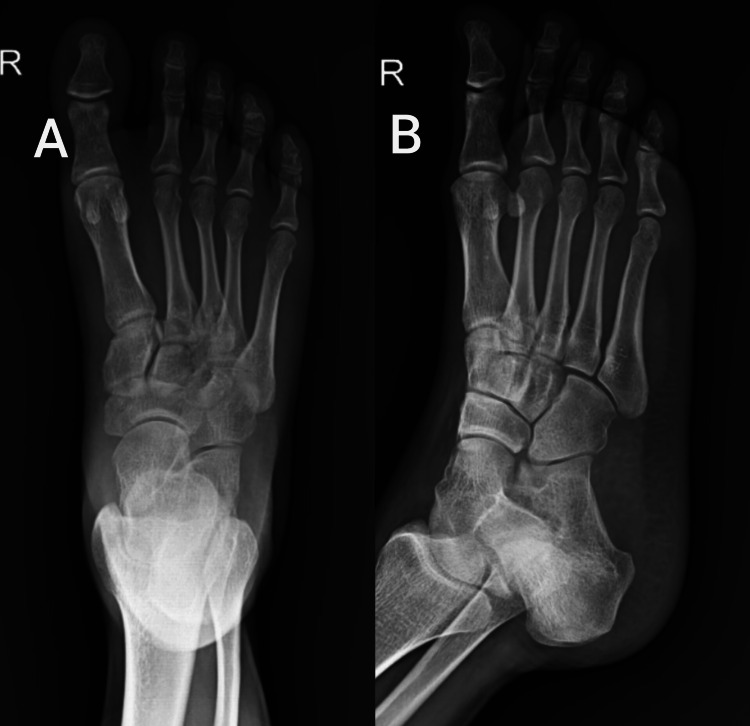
X-ray of the right ankle joint A: anteroposterior view, B: lateral view
